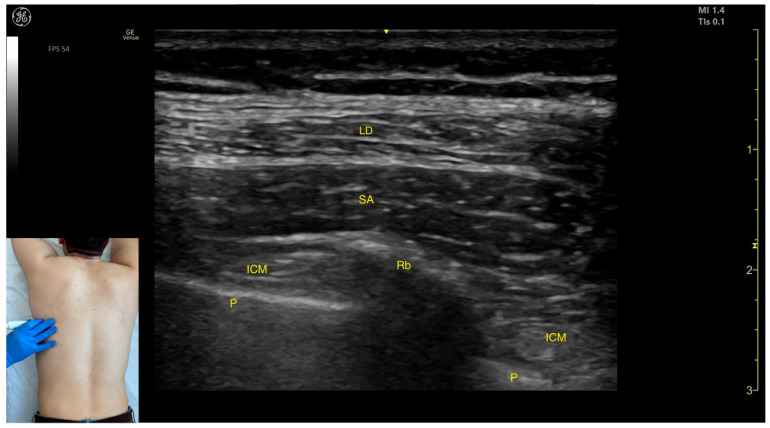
Ultrasound anatomy of the latissimus dorsi (LD) and serratus anterior (SA) with key landmarks: LD—latissimus dorsi; SA—serratus anterior; ICM—intercostal muscle; Rb—rib; and P—pleura.
